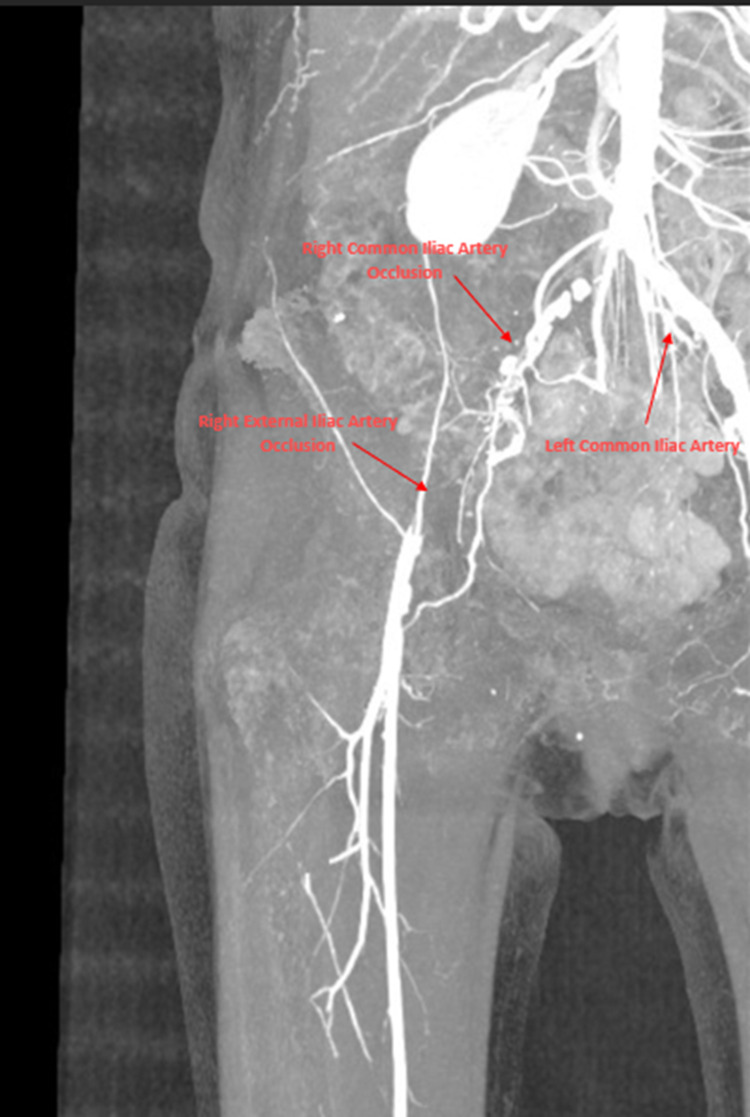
CTA of the abdominal aorta with runoff showing occlusion of the right common and right external iliac arteries. CTA: computed tomography angiography
